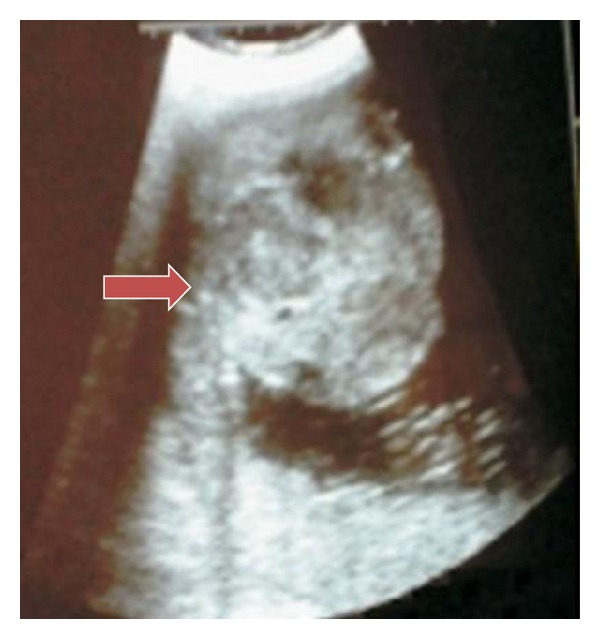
Transabdominal ultrasound showing echogenic large lobular mass suggestive of chorioangioma measuring approximately 12 × 12 cm, arrow pointing to tumor.
